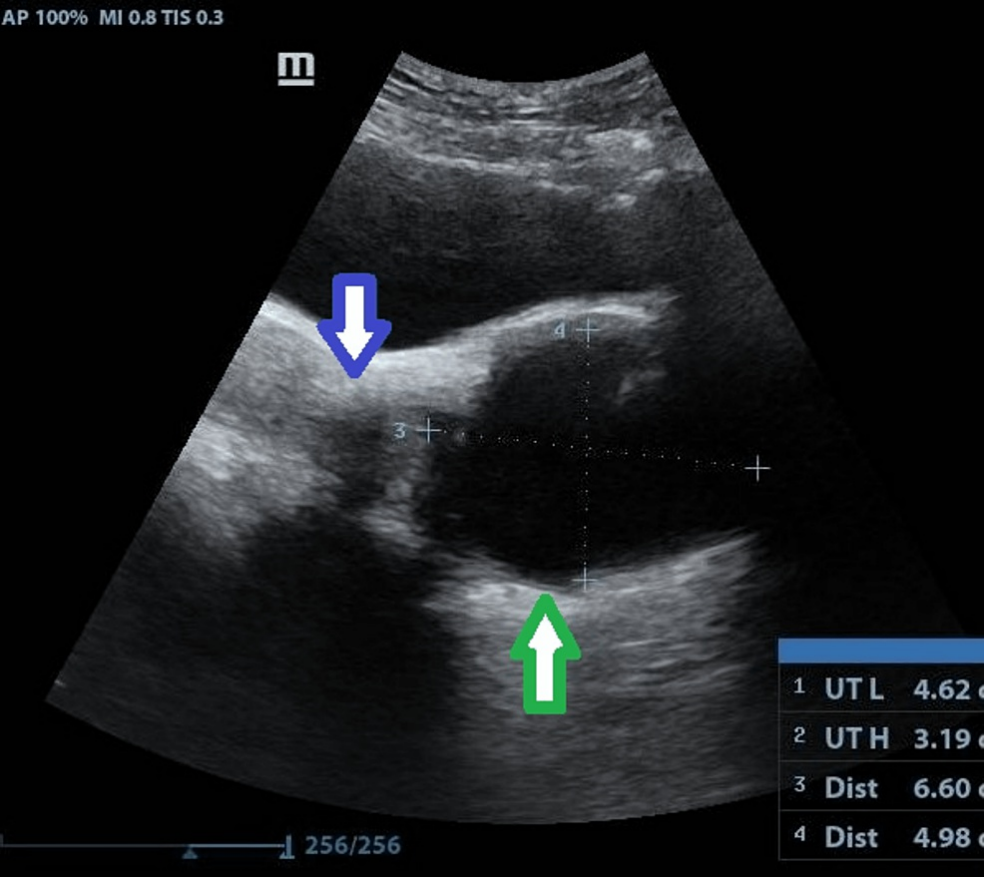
Ultrasonography shows an anechoic cyst (green arrow mark) of size 6.6 x 4.9 cm distal to the cervix (blue arrow mark) in the vaginal cavity
